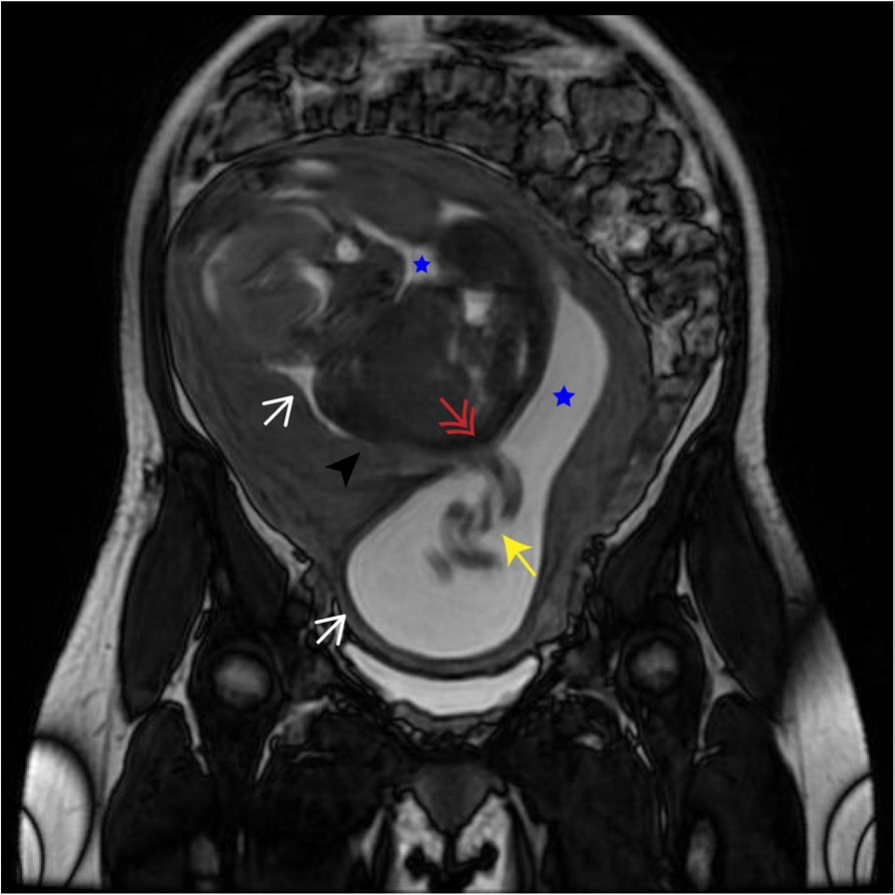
Magnetic resonance images. The amniotic sac lying across two hemicavities (white arrows) by a 10 mm defect (red double arrow) at the septum. The fetus (black arrow head) with little amniotic fluid (blue pentagram) in the right hemicavity and the umbilical cord (yellow arrow) with most amniotic fluid in the left hemicavity
